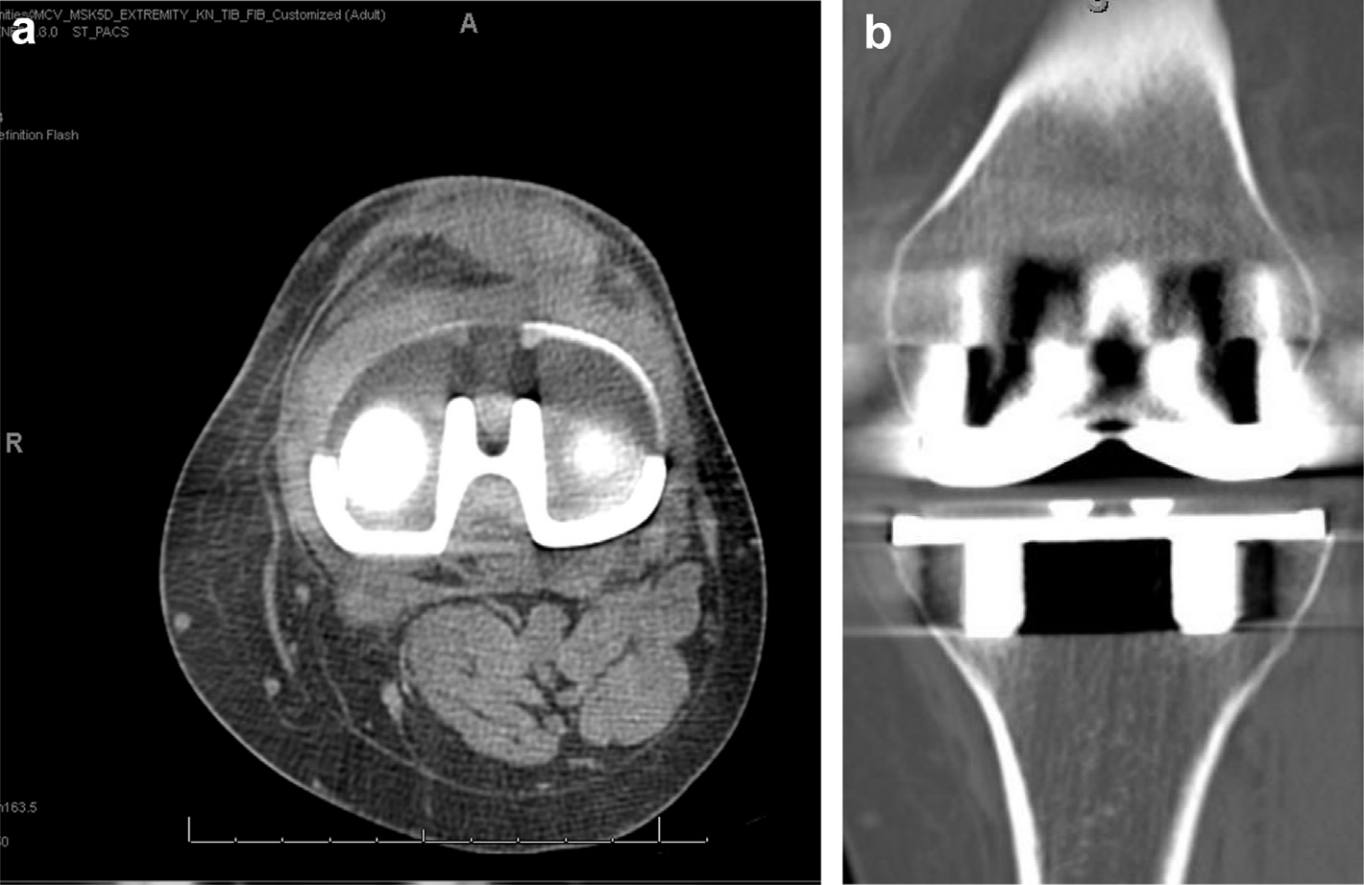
(a) Axial CT scan demonstrating intact tibial baseplate. (b) Coronal CT scan demonstrating intact tibial baseplate.
